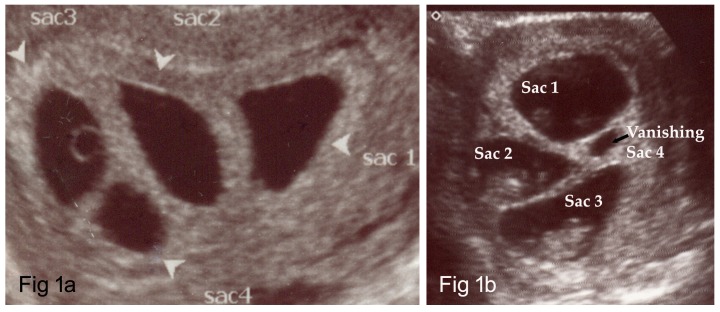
An ultrasound image showing four gestational sacs (Fig 1a) and a vanishing sac 4 (Fig 1b)
